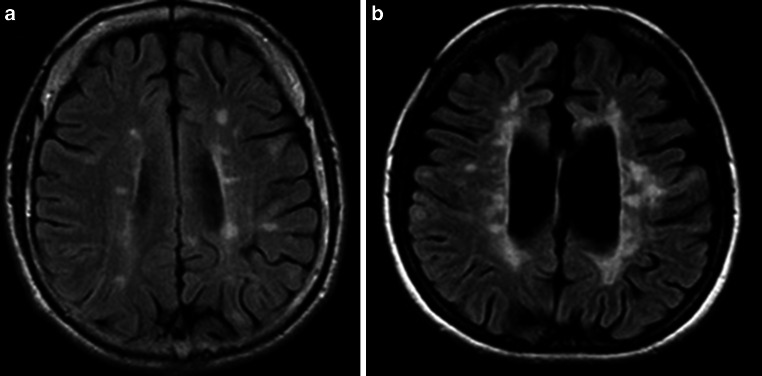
Brain MRI scans in PPMS patients who are similar to those seen in relapse–remitting multiple sclerosis (RRMS) and secondary progressive multiple sclerosis (SPMS). Brain MRI scans of PPMS patients with different MRI presentations a FLAIR image, axial plane. Multiple focal lesions were located in the periventricular, deep and juxtacortical white matter. This MRI is similar to a typical MRI scan observed with RRMS. b Fluid attenuated inversion recovery (FLAIR) image, axial plane. Focal and confluent white matter lesions were located in the periventricular white matter; some lesions located in the juxtacortical regions were similar to those seen in SPMS. Signs of brain atrophy are also visible
